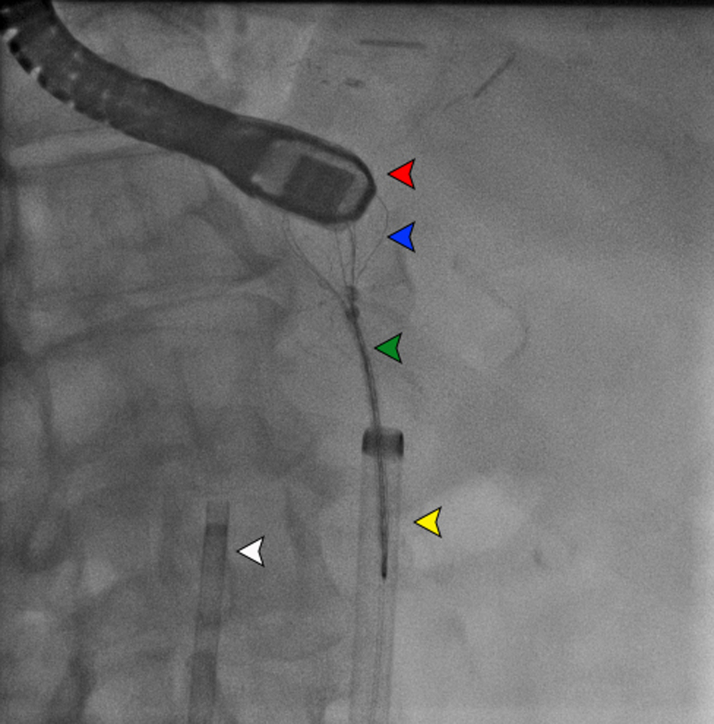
Fluoroscopic Image Showing Aortic Snare Removal of the Embolized Watchman Device The red arrowhead indicates the TEE probe; the blue arrowhead indicates the embolized Watchman device captured by snare; the green arrowhead indicates the triple-loop snare; the yellow arrowhead indicates the 16-F aortic sheath; and the white arrowhead indicates the ThermoCool ablation sheath. TEE = transesophageal echocardiography.
